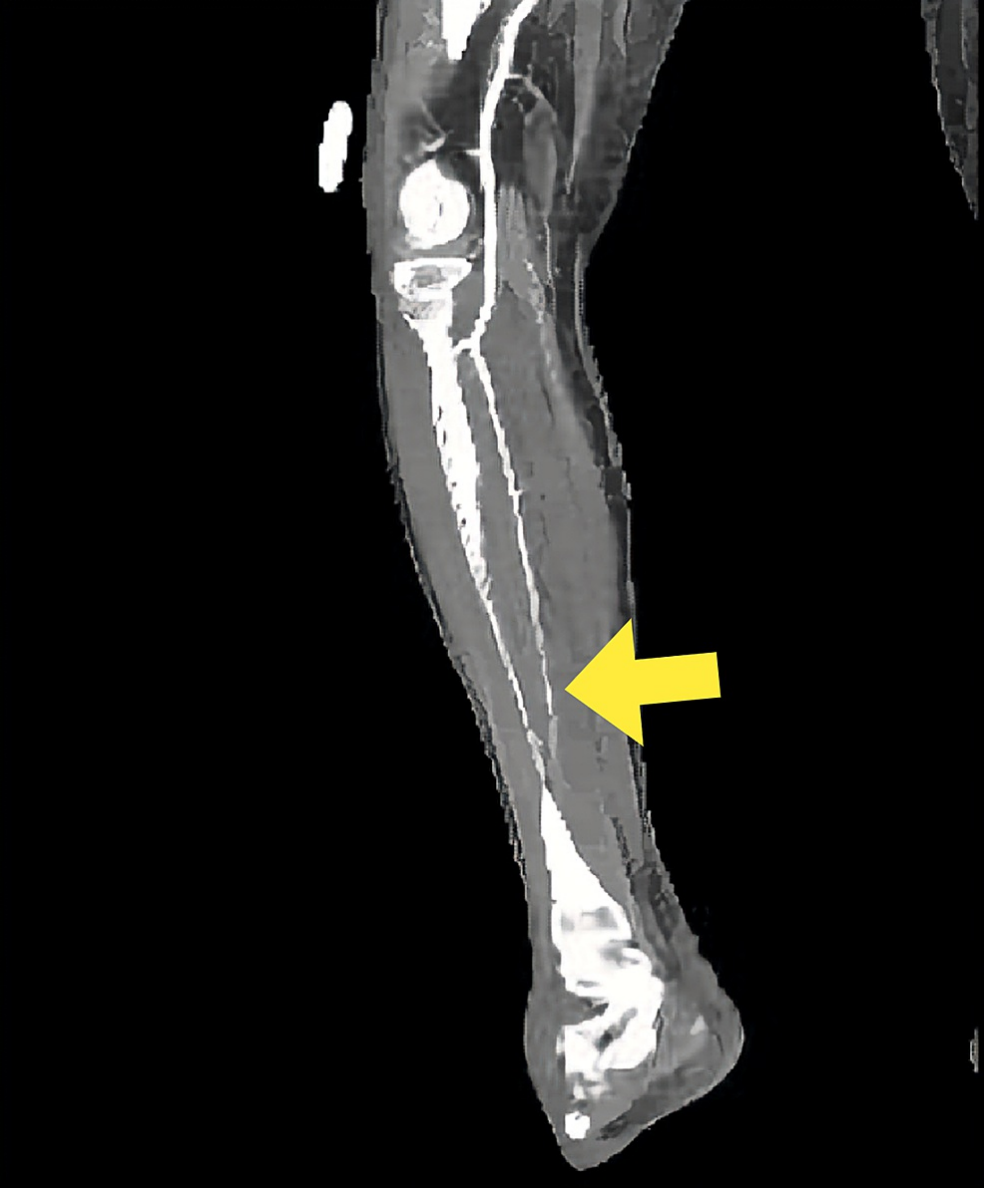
Computed tomography of the right lower extremity showing a tapered distal occlusion of all three blood vessels
